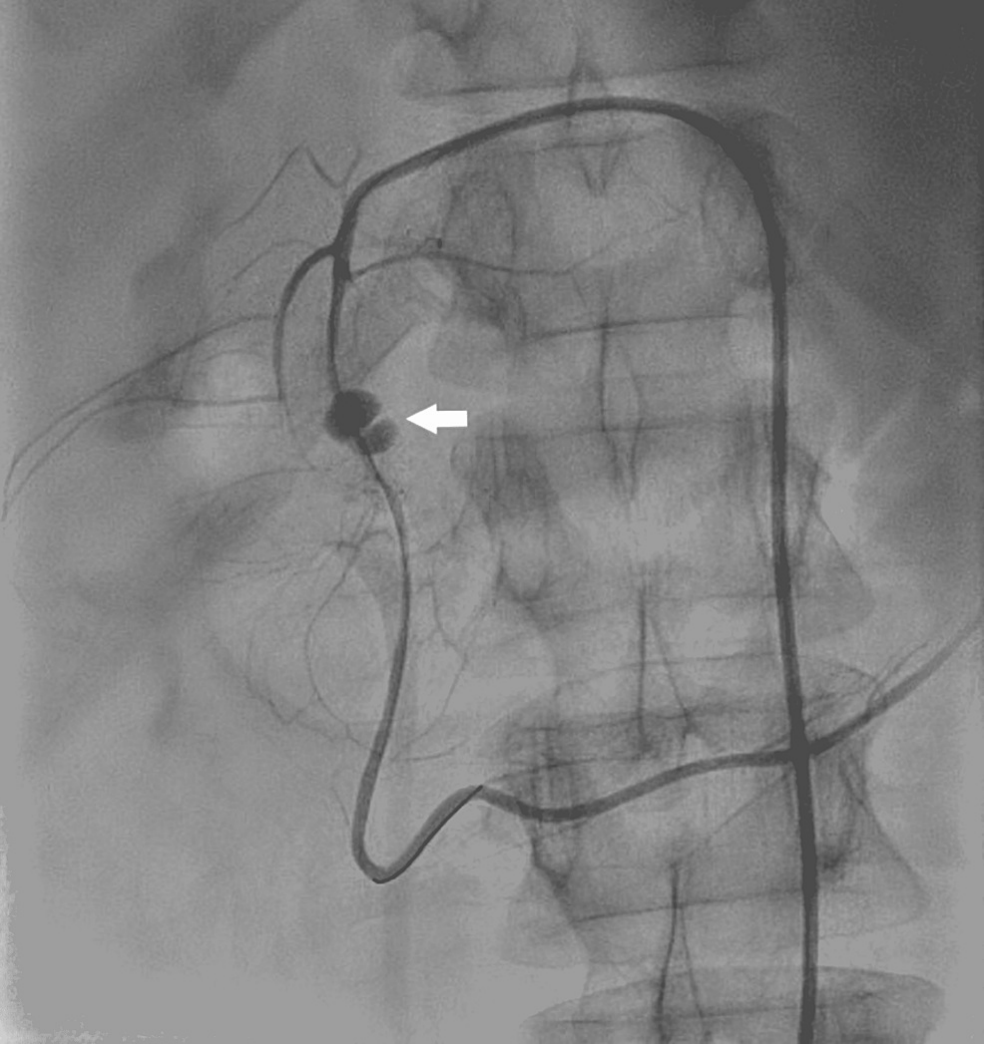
CT angiography via the right femoral route CT: Computed Tomography The arrow shows a superior pancreaticoduodenal artery pseudoaneurysm.
